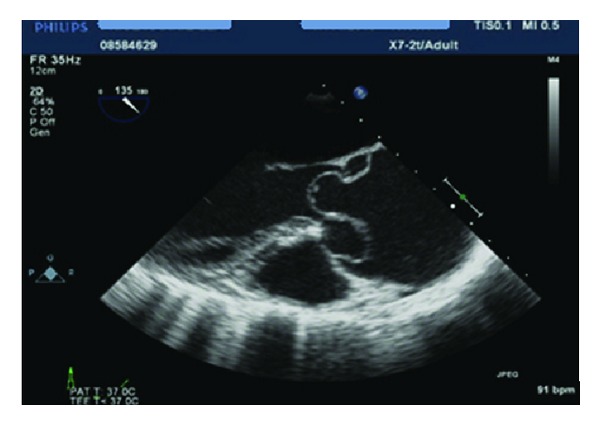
Transoesophageal echocardiogram showing Type A aortic dissection with effacement of the sinotubular junction.
